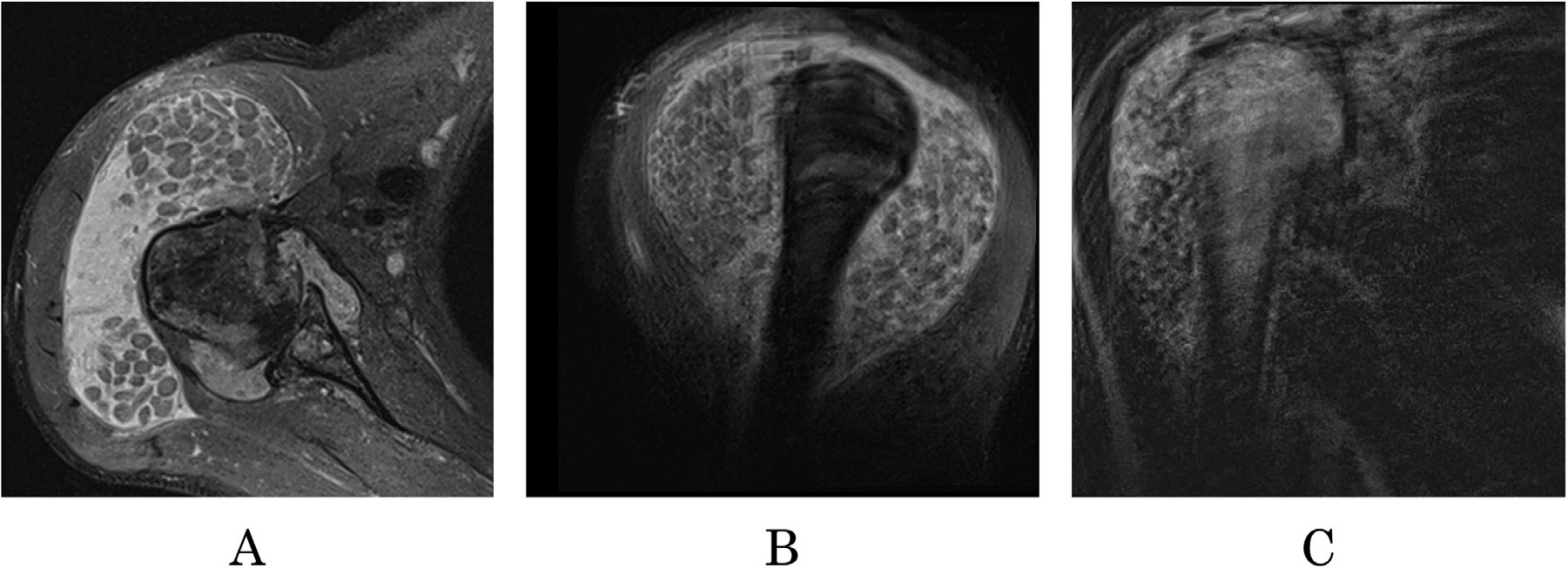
In Figure 1A, an axial view of PD_FS_TSE sequences shows a tumor formation in the right shoulder. In 2A, a coronal view of the SS in PD MRI. 2C, the PD_FS_TSE shows the sagittal view of the neoplasm.
